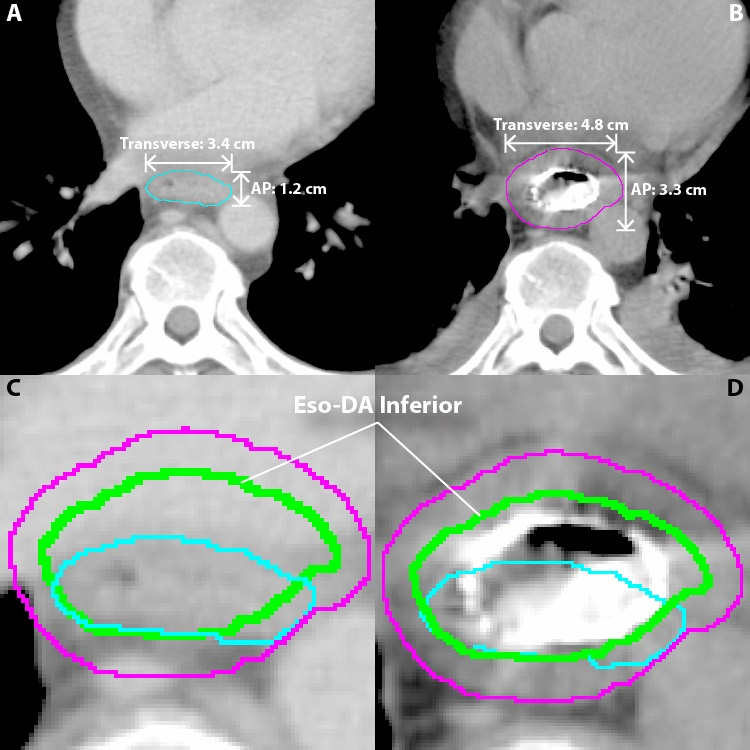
Diameter approximation (DA) structures. The DA esophageal volume (green) is based on the difference in the AP and transverse measurements of the pre‐ (cyan) and post‐ (magenta) InfCyl structures. For the DA method, width of the pre‐ and post‐stent InfCyl (shown) and SupCyl (not shown) volumes were measured in the anterior/posterior (AP) and transverse directions on the superior, middle, and inferior slices of each respective volume. An average diameter difference for each of the three values was calculated for both the AP and transverse dimensions. The post‐stent SupCyl and InfCyl AP and transverse diameters were then altered by their respective differences to create new esophageal volumes, Eso‐DA Superior (not shown) and Eso‐DA Inferior (shown), to ultimately make a new PTV (PTV‐DA) (not shown). Axial CT (a) showing the AP and transverse measurements of the pre‐stent SupCyl structure (cyan). Axial CT (b) showing the AP and transverse measurements of the post‐stent SupCyl structure (magenta). For this patient on this slice stent placement increased the AP dimension by 2.1 cm and the transverse dimension by 1.4 cm. Pre‐stent CT axial section (c) now showing the Eso‐DA Inferior structure (green). Post‐stent CT axial section (d) now showing the Eso‐DA Inferior structure (green). InfCyl pre‐stent (cyan); InfCyl post‐stent (magenta); Eso‐DA Inferior (blue); Eso‐VA Inferior (green).
